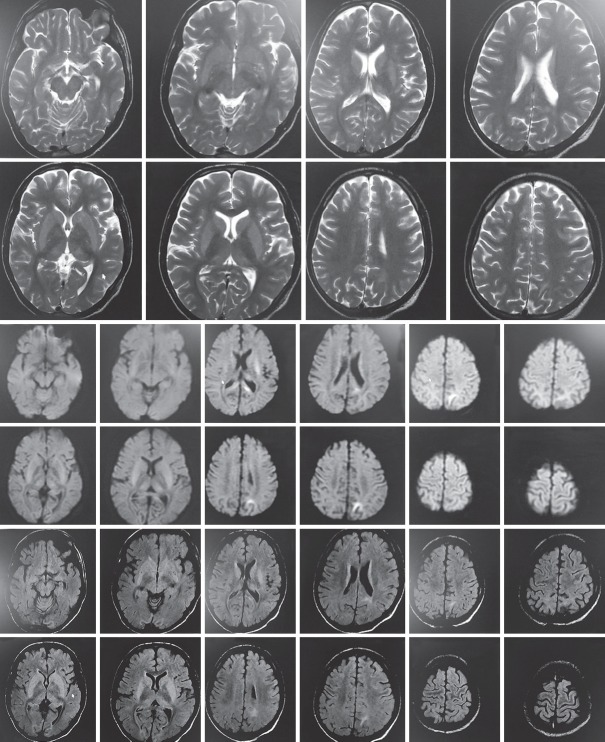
There is presence of fairly bilaterally symmetrical, ill defined, mild T2, and FLAIR hyperintensity in bilateral basal ganglia and thalamus. In the splenium of corpus callosum and in the cortical–subcortical aspect of bilateral occipital lobes and left posterior parietal lobe there is mild DWI hyperintensity with corresponding mild hypointensity on ADC images (predominantly in the splenium of corpus callosum and in subcortical aspect of left posterior parietal lobe), suggesting diffusion restriction.
